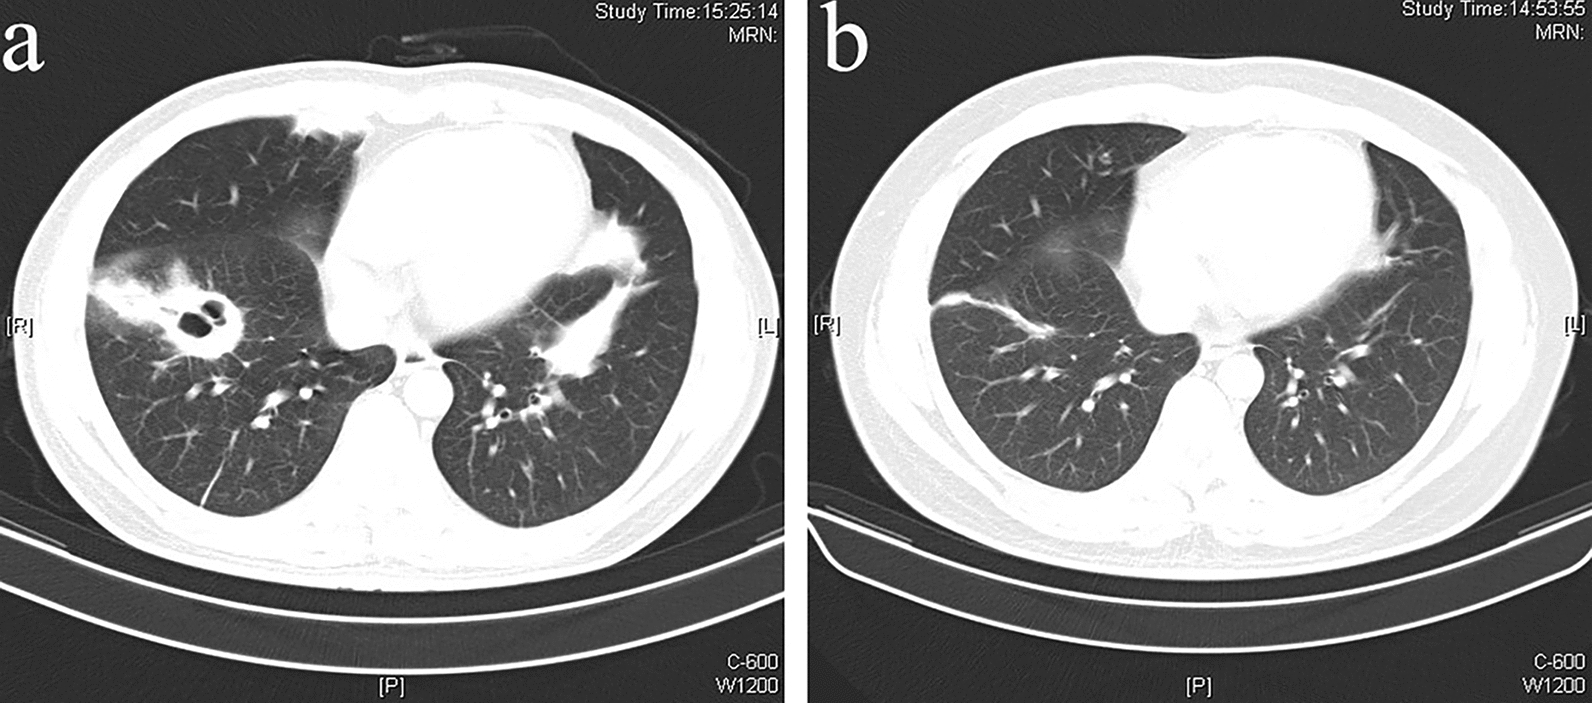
a Axial image from CT of the chest demonstrating bilateral patchy consolidation with pulmonary cavitation formation. b A follow-up CT scan after 6 months of antifungal treatment indicated the lesions nearly resolved
